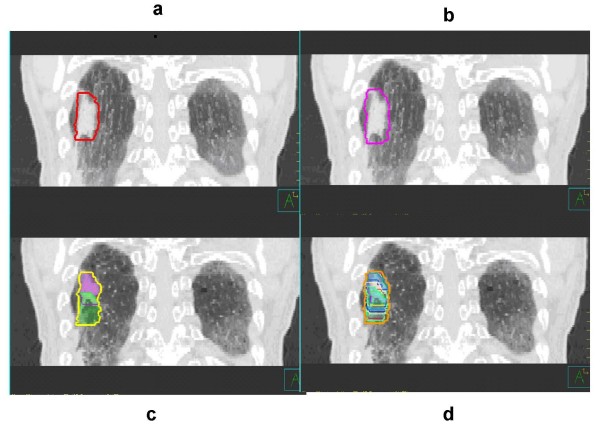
Delineation of IGTV for stage I lung tumors based on (a) IGTV_MIP_, (b) IGTV_MIP-Modified_, (c) IGTV_2Phases_, and (d) IGTV_AllPhases _of a 4-D CT data set. MIP-based contours, as shown in panels (a) and (b), are as they appear on the MIP data set. Phase-based contours, as shown in panels (c) and (d), are registered to the peak exhalation phase of the 4-D CT data set.
